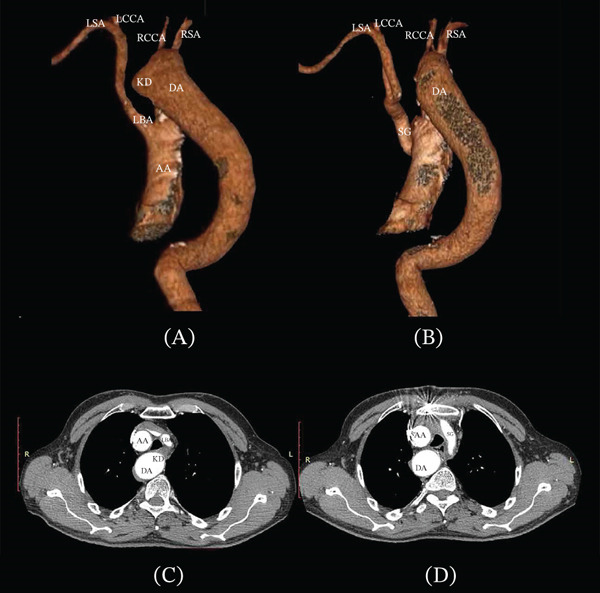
Posterior view of 3D computed tomography (CT) (A) before and (B) after the procedure. Arterial phase of the contrast‐enhanced CT scan in an axial view (C) before and (D) after the procedure. AA, ascending aorta; DA, descending aorta; ES, esophagus; IV, innominate vein; KD, Kommerell’s diverticulum; LBA, left brachiocephalic artery; LCCA, left common carotid artery; LSA, left subclavian artery; SG, synthetic graft; RCCA, right common carotid artery; RSA, right subclavian artery; TR, trachea.
